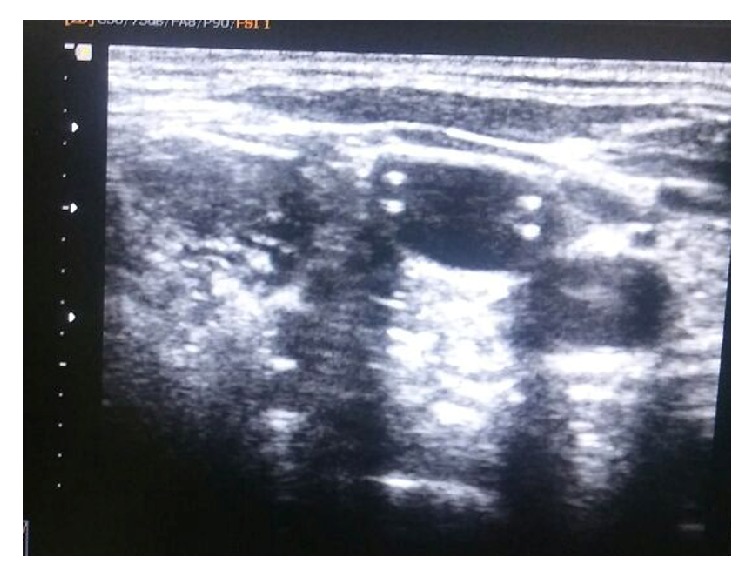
The right internal jugular vein is clearly evident on ultrasonography. Two lumens of the catheter can also be seen.
